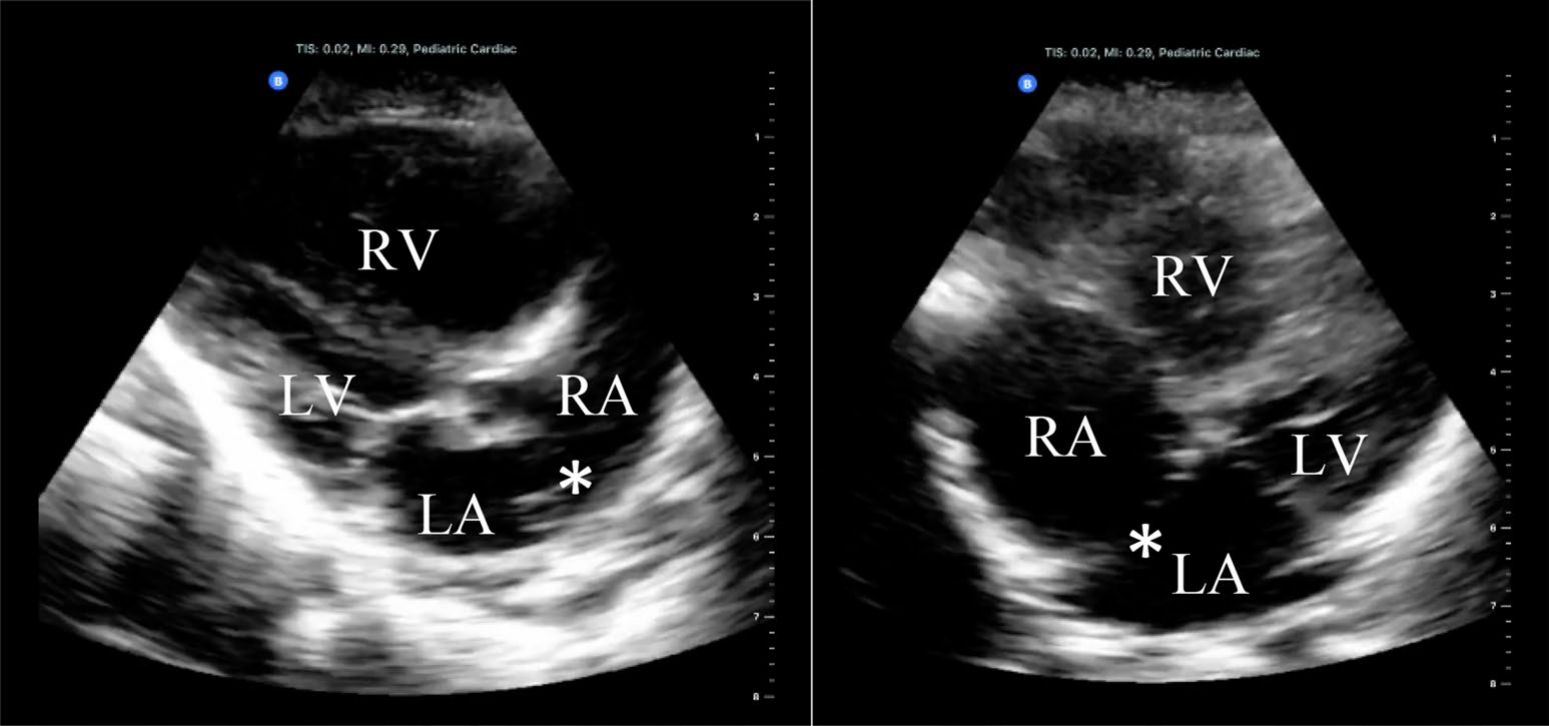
3-month-old male presenting with acute respiratory distress. POCUS revealed a dilated right atrium and ventricle with a prominent atrial septal defect, seen in parasternal long view (left) and a slightly modified apical four chamber view (right). LA, Left Atrium, LV, Left Ventricle, RA, Right Atrium, RV, Right Ventricle, *, Atrial Septal Defect
